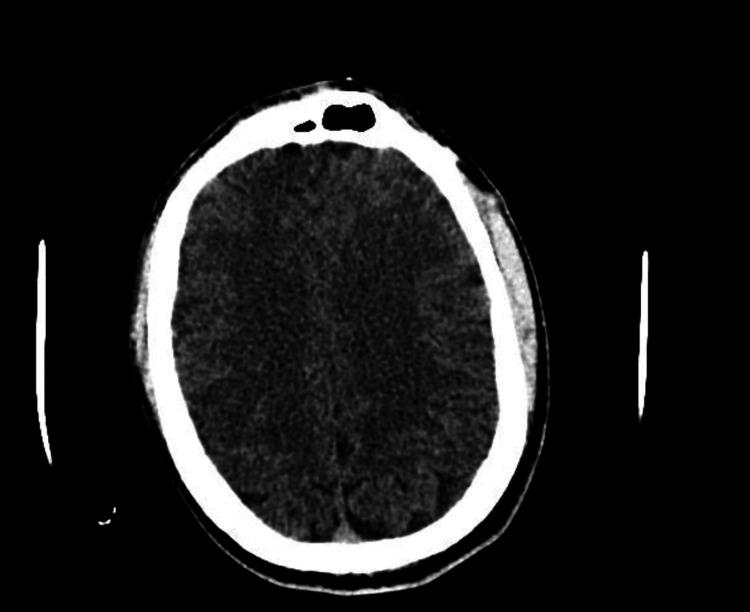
Head CT scan showing no abnormalities. CT scan of the skull and brain was performed using a multidetector helical technique. No contrast medium was administered.
